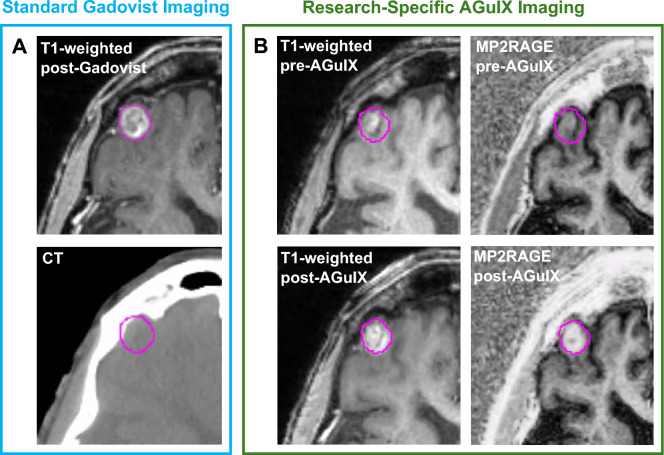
The propagation of tumor delineation from the planning scans to the MP2RAGE output. (A) A $${T}_{1}$$-weighted scan taken after the administration of Gadovist and the CT scan it was then registered to for treatment planning purposes. (B) Both MPRAGE ($${T}_{1}$$-weighted) and MP2RAGE scans before and after the administration of AGuIX. In each instance, the tumor delineation was propagated from the planning scans to the research specific scans by image registration.
